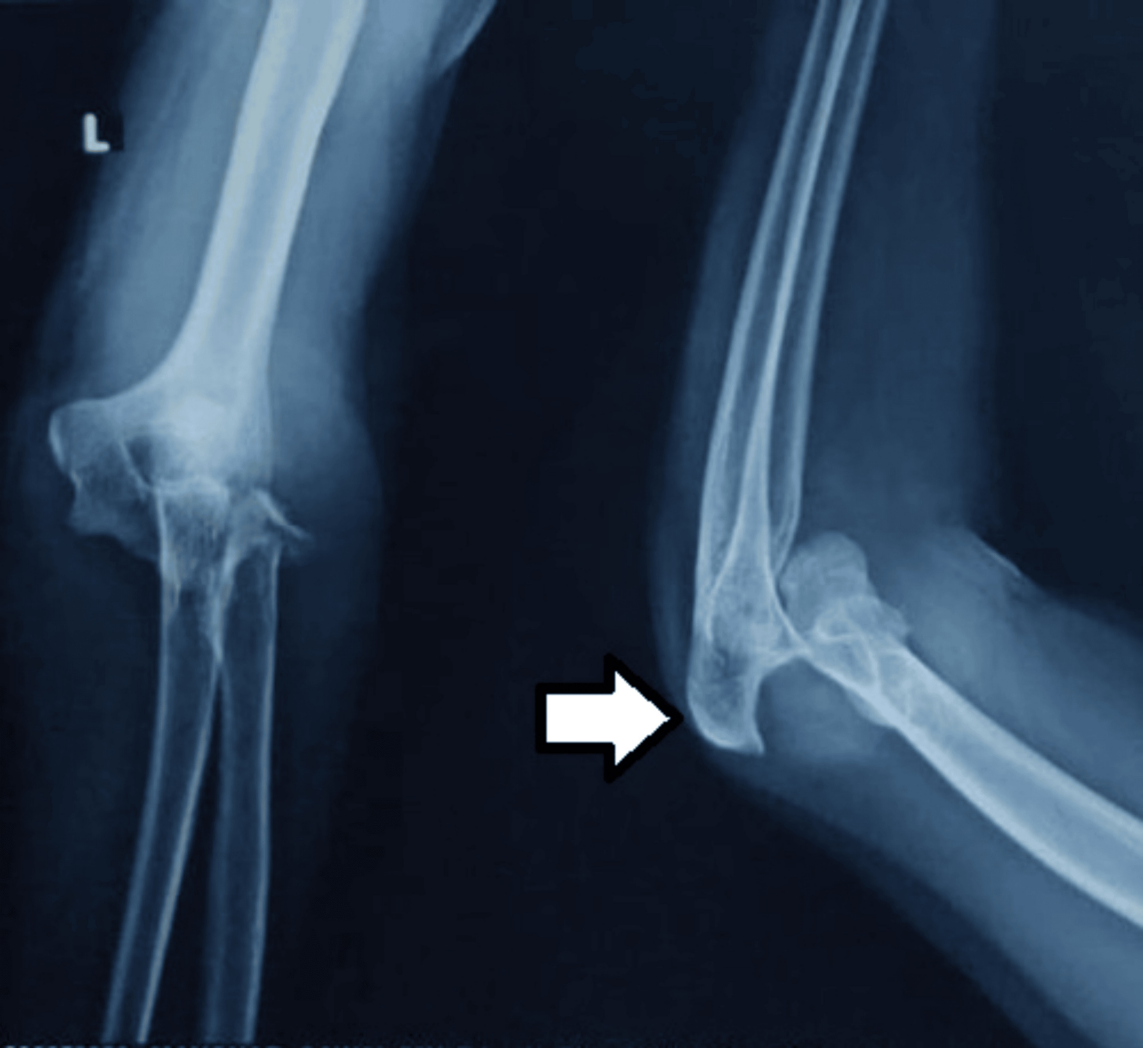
Prereduction AP and lateral radiographs showing elbow dislocation (white arrow) AP: Anteroposterior
